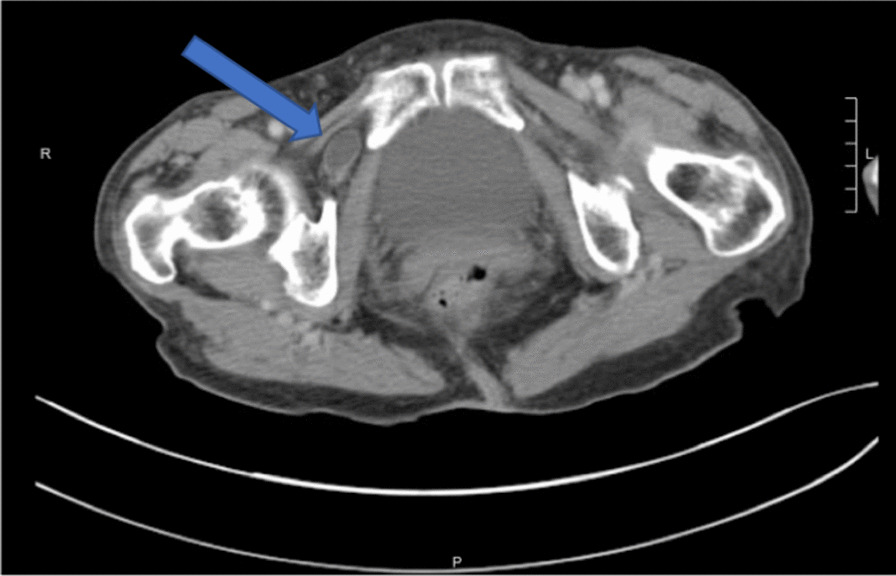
Axial computed tomography image showing a right-sided obturator hernia (denoted by the blue arrow)
